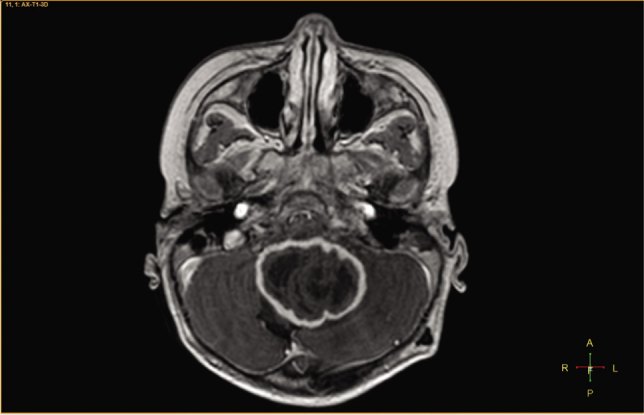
Axial T1-weighted image with contrast agent reveals a giant ring-enhancing mass.
